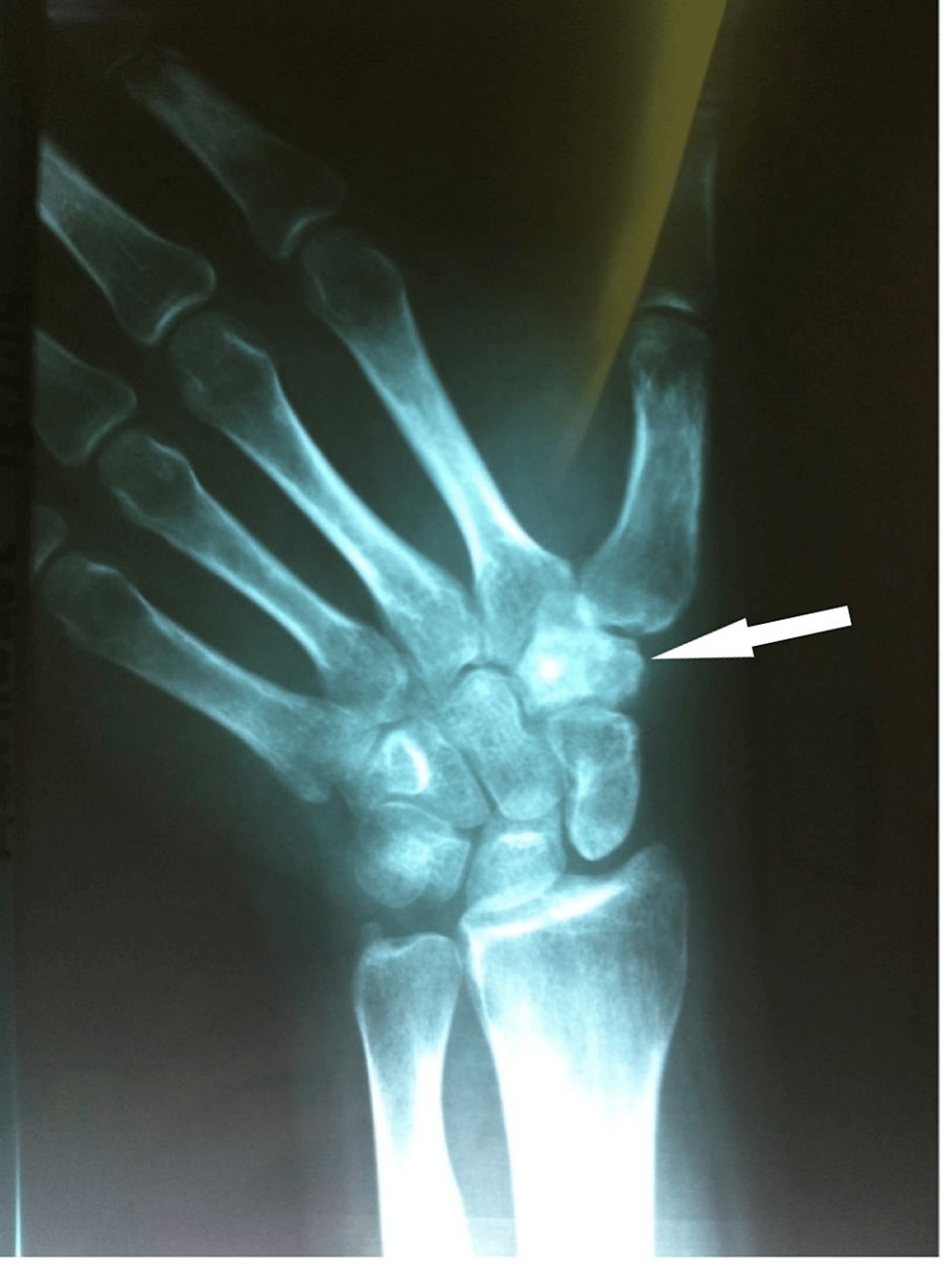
Initial radiograph Anteroposterior radiograph of the left wrist showing bone sclerosis of the trapezium.
